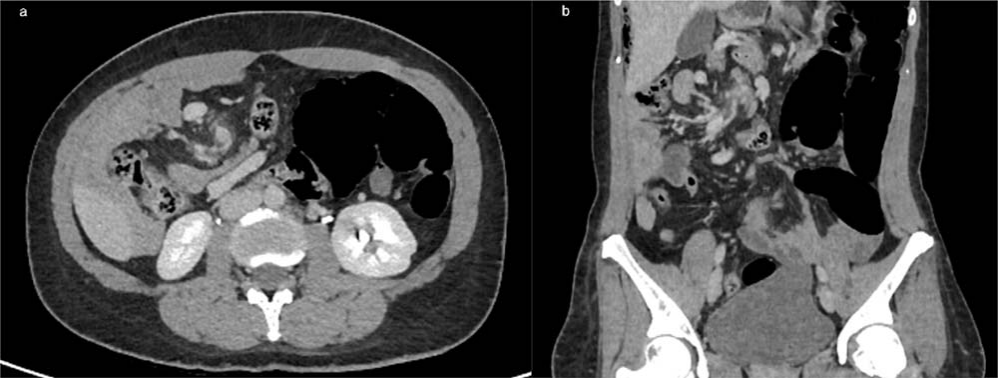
(a) Axial contrast-enhanced CT showing the classic ‘whirlpool sign,’ with clockwise swirling of the superior mesenteric vein and mesenteric fat around the SMA, consistent with midgut volvulus (b) coronal CT reconstruction confirming mesenteric torsion.
